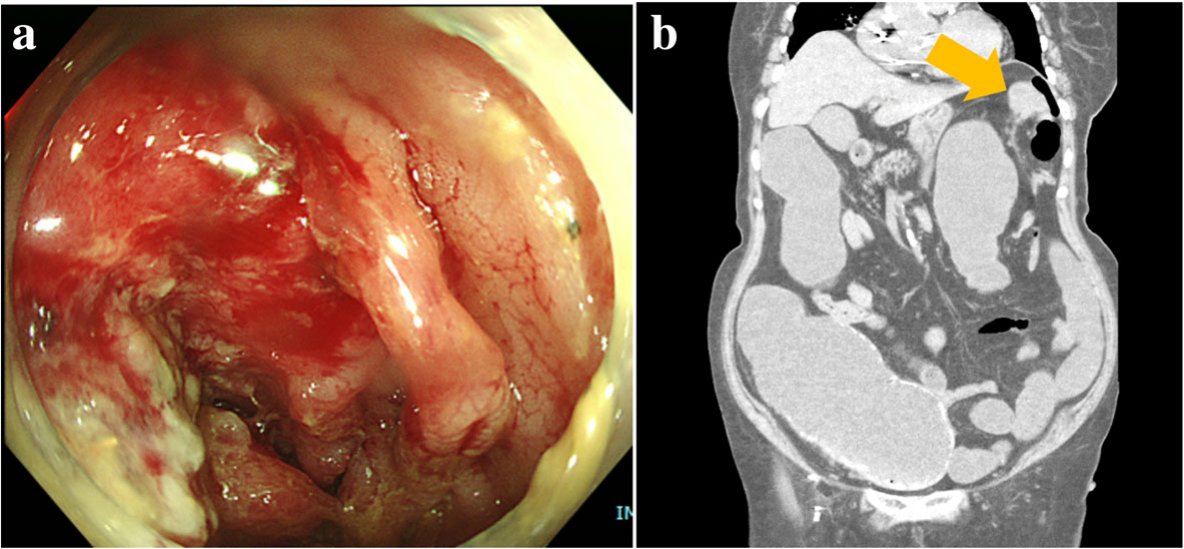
Preoperative colonoscopy and computed tomography findings. a Colonoscopy confirmed descending colon cancer and obstruction. b Computed tomography revealed an obstructive ileus due to descending colon cancer (yellow arrow)
